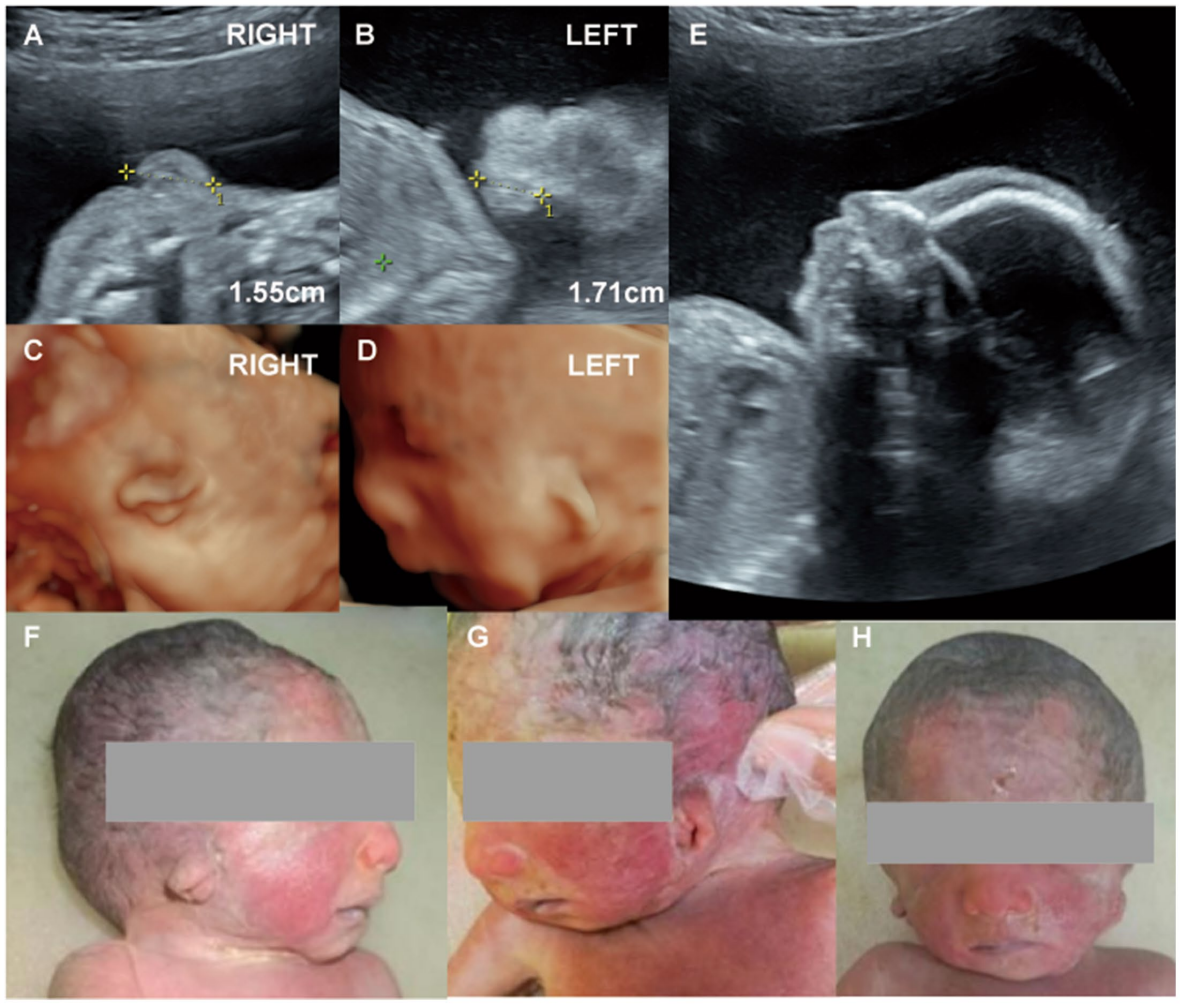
(Case 2). (A,B) At 23 weeks’ gestation, routine ultrasound revealed bilateral microtia. (C,D) Three-dimensional imaging showed that both ears were small, misshapen, and low-set, with rotation of the right ear. (E) Additional findings included micrognathia, a single umbilical artery, and polyhydramnios. Genetic testing was normal. (F-H) Labor was induced at 26 weeks, and postnatal examination confirmed bilateral Grade II microtia.
